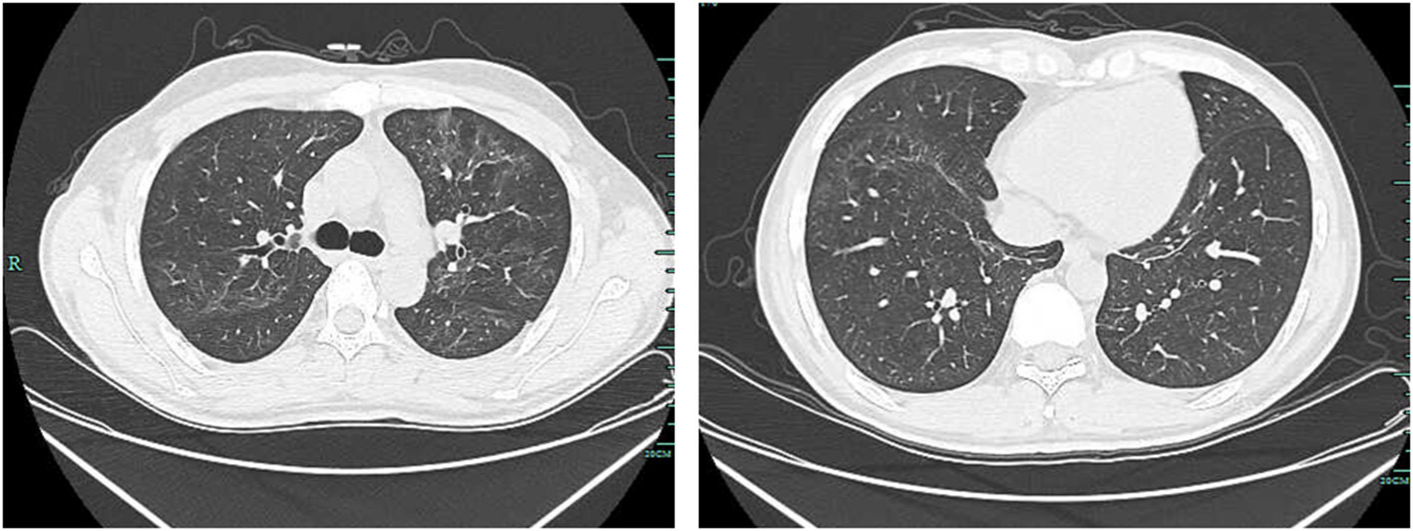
CT of the lung showed that multiple infections occurred in bilateral lungs, and they were better, slightly diffuse, and with a ground glass-like high density of shadows on day 12.
